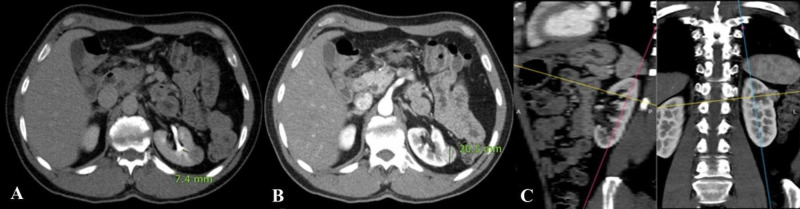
Low complexity lesion (5p) according to the RENAL nephrometry score A) Excretory phase of a triphasic CT scan showing left renal cell carcinoma, which is 7.4 mm away from the renal collecting system. B) Arterial phase contrast-enhanced CT scan showing the maximum diameter of the lesion measuring 20.5 mm. C) Sagittal and coronal reformatted sections showing the lesion location entirely in the upper pole. RENAL: radius, exophytic/endophytic properties, nearness of tumor to the collecting system or sinus in millimeters, anterior/posterior, location relative to polar lines
